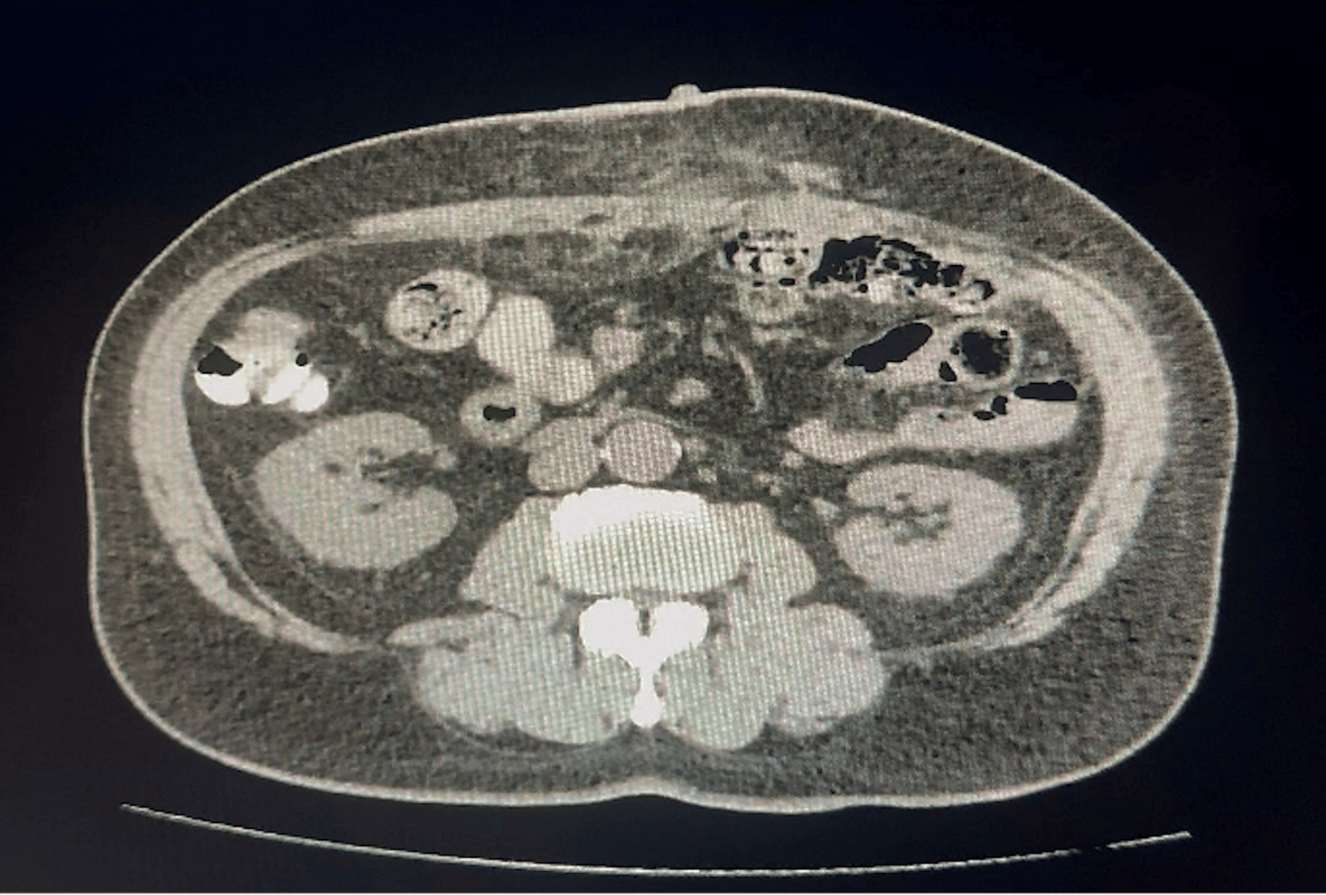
Abdominal CT scan at one month postoperatively.
